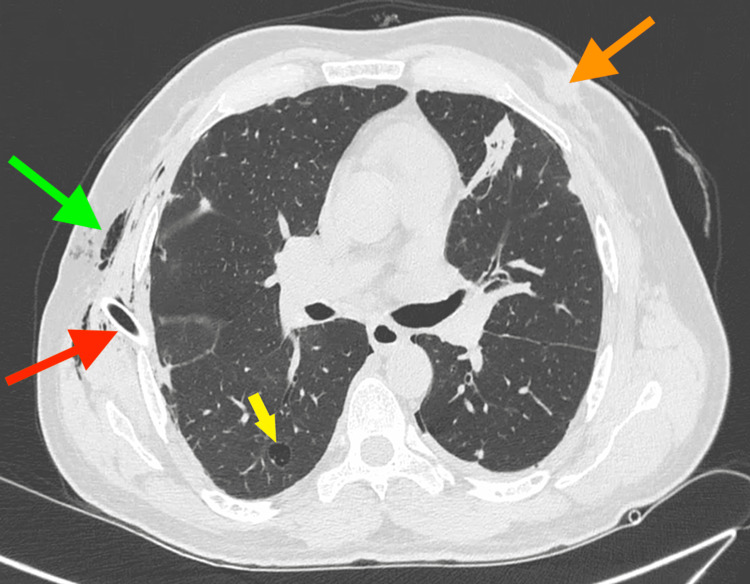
CT Chest image after receiving chemotherapy showed cystic change in the parenchymal nodule (yellow arrow), with right-sided surgical emphysema (green arrow) and right-sided ICT in situ (red arrow). A lesion can also be seen in the left breast (orange arrow).
